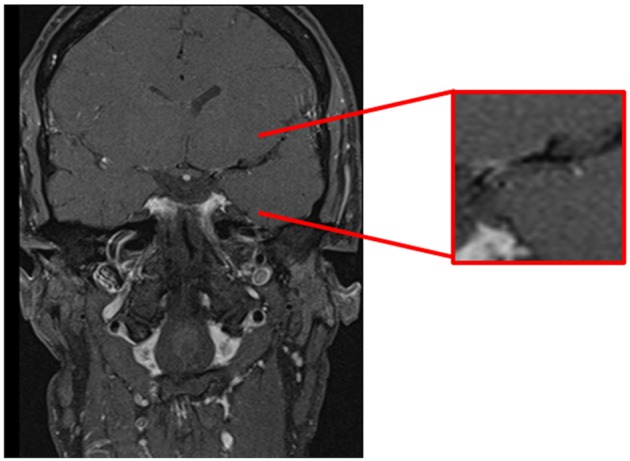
Cranial MRI, coronal T1-weighted-(dark-blood) sequence demonstrating contrast enhancement of the vessel wall in the first segment of the left middle cerebral artery.
